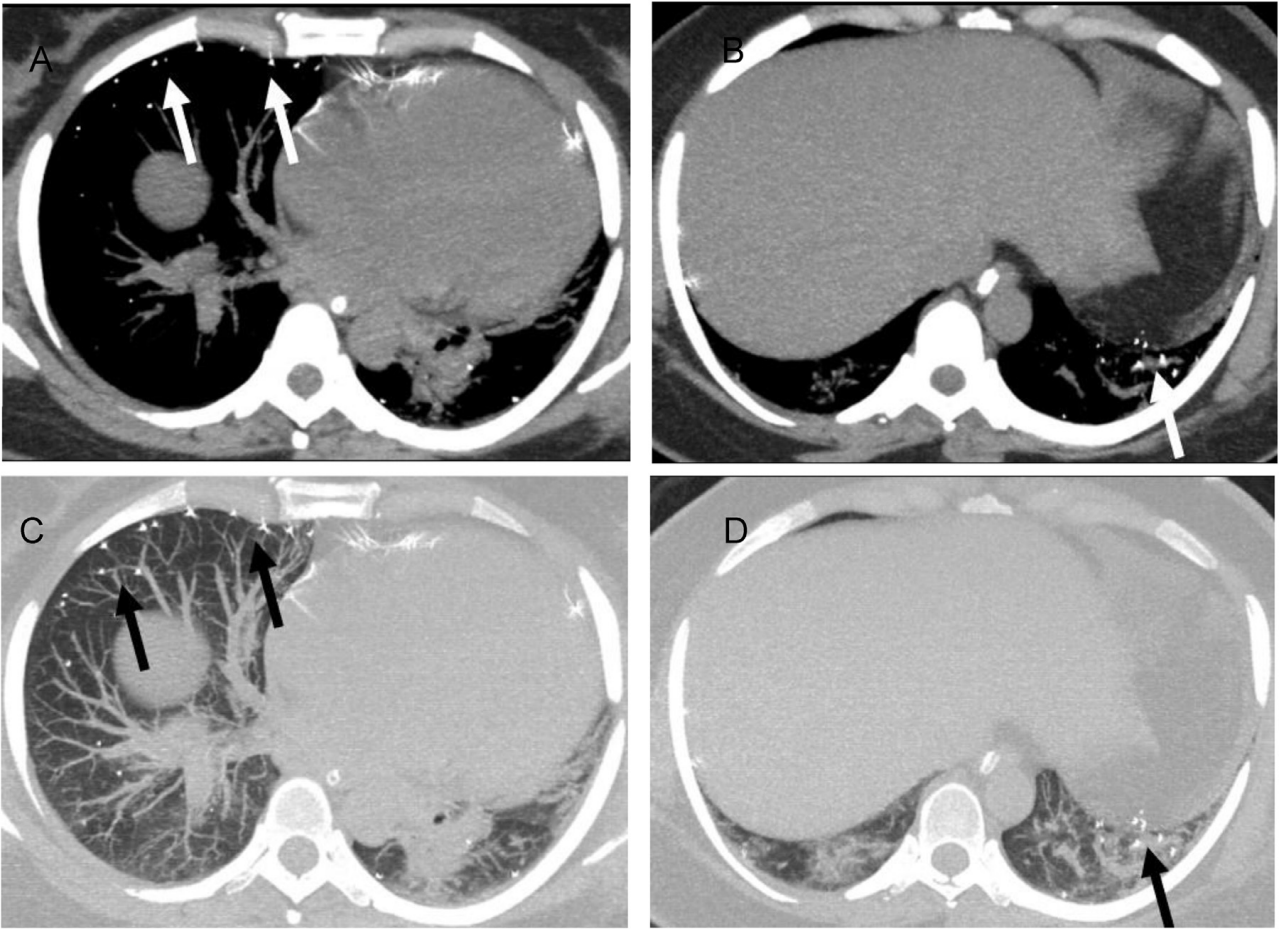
Chest axial CT scan in MIP reconstruction. (A and B) The mediastinal window and (C and D) the lung window shows some hyperdense, rounded, and oval nodules in the lung parenchyma closely related to the pulmonary arteries. There are also similar foci in the right ventricle.
